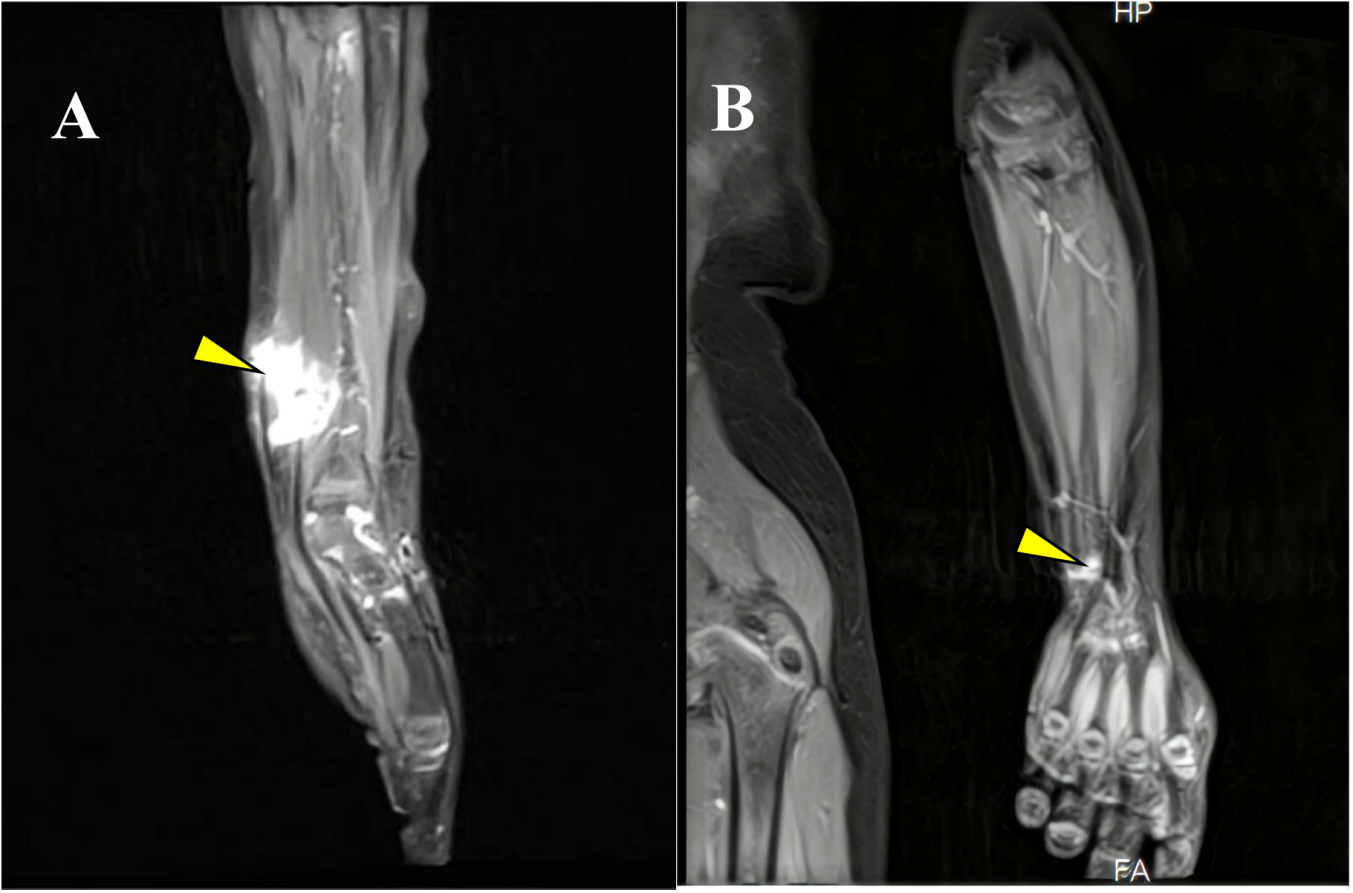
(A) MRI highlighting the tumor in the left wrist at initial presentation. (B) Follow-up MRI demonstrating the post-treatment status of the left wrist lesion (yellow arrow).
